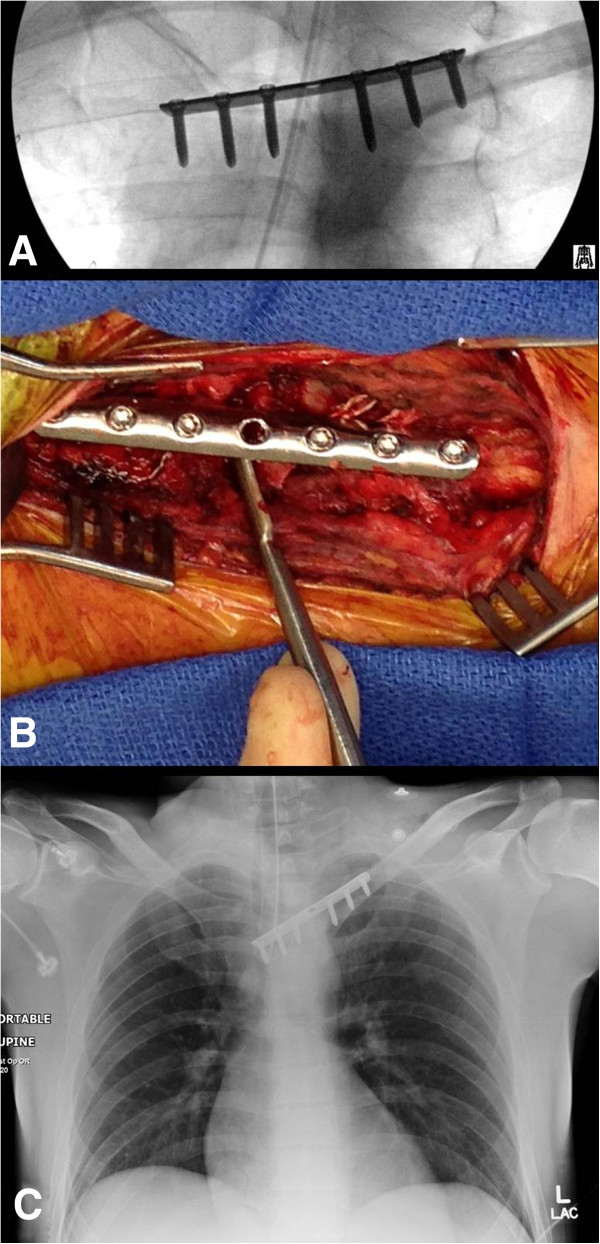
Bridge plating of the left sternoclavicular joint using a 3.5 mm third-tubular locking plate. Panel A shows the intraoperative fluoroscopic view after bridge plate fixation. The periosteal elevator in panel B denotes the sternoclavicular joint space below the plate. An on-table chest radiograph was obtained to rule out an iatrogenic left-side pneumothorax (C).
